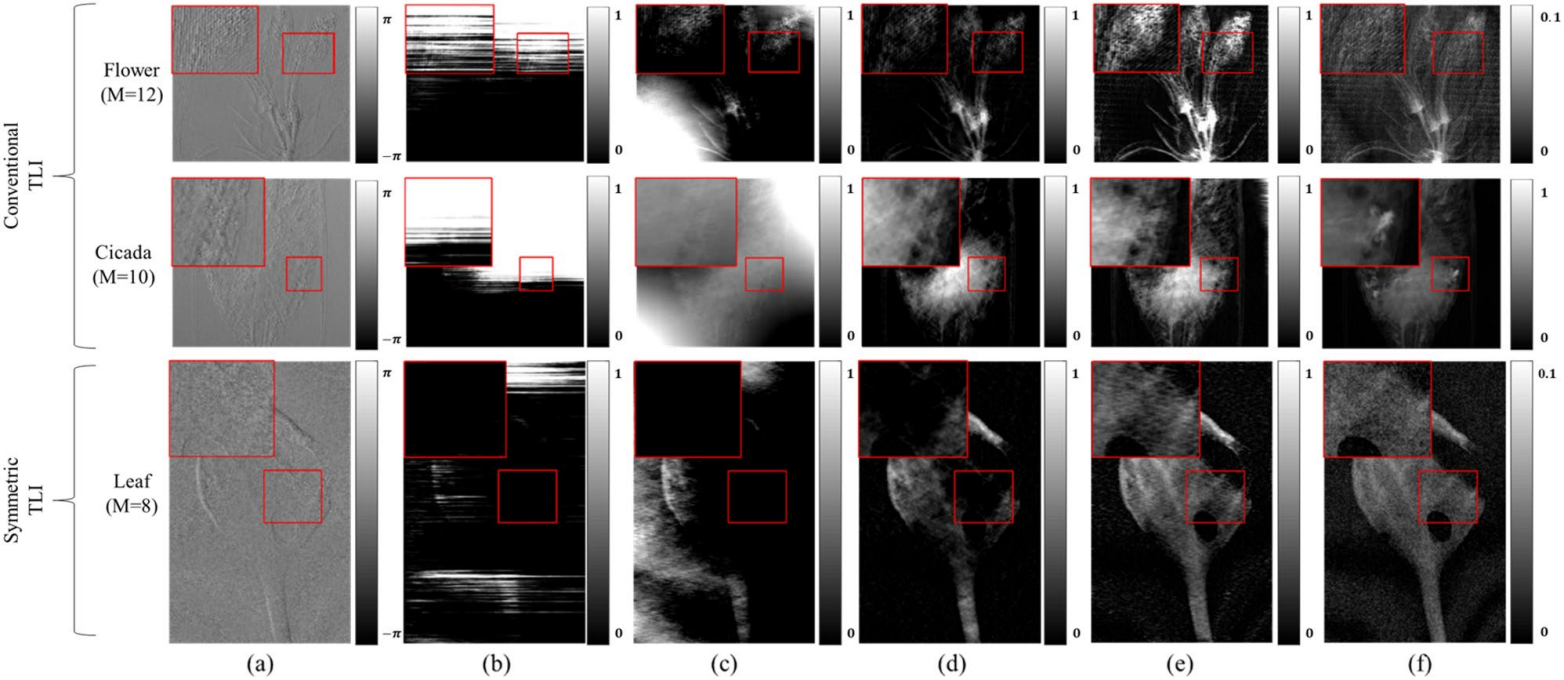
The X-ray grating interferometer comparison results. (a) DPCI, (b) direct integration, (c) WF filtering, (d) iterative method, (e) the proposed method, (f), transmission. The display windows are same for (b–e), and the display window of the first and third images of (f) are [0, 0.1], and the second is [0, 1], respectively. The variable M denotes the total phase stepping number. The red rectangle regions are selected ROIs.
